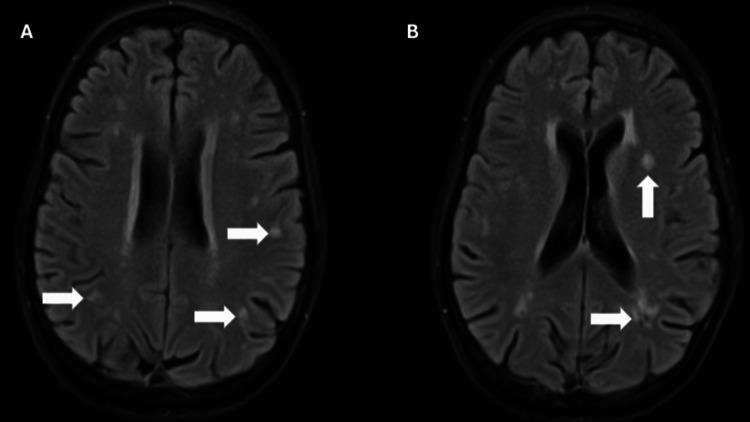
T2 fluid-attenuated inversion recovery (FLAIR) sequence MRI demonstrating multiple subcortical hyperintensities.
